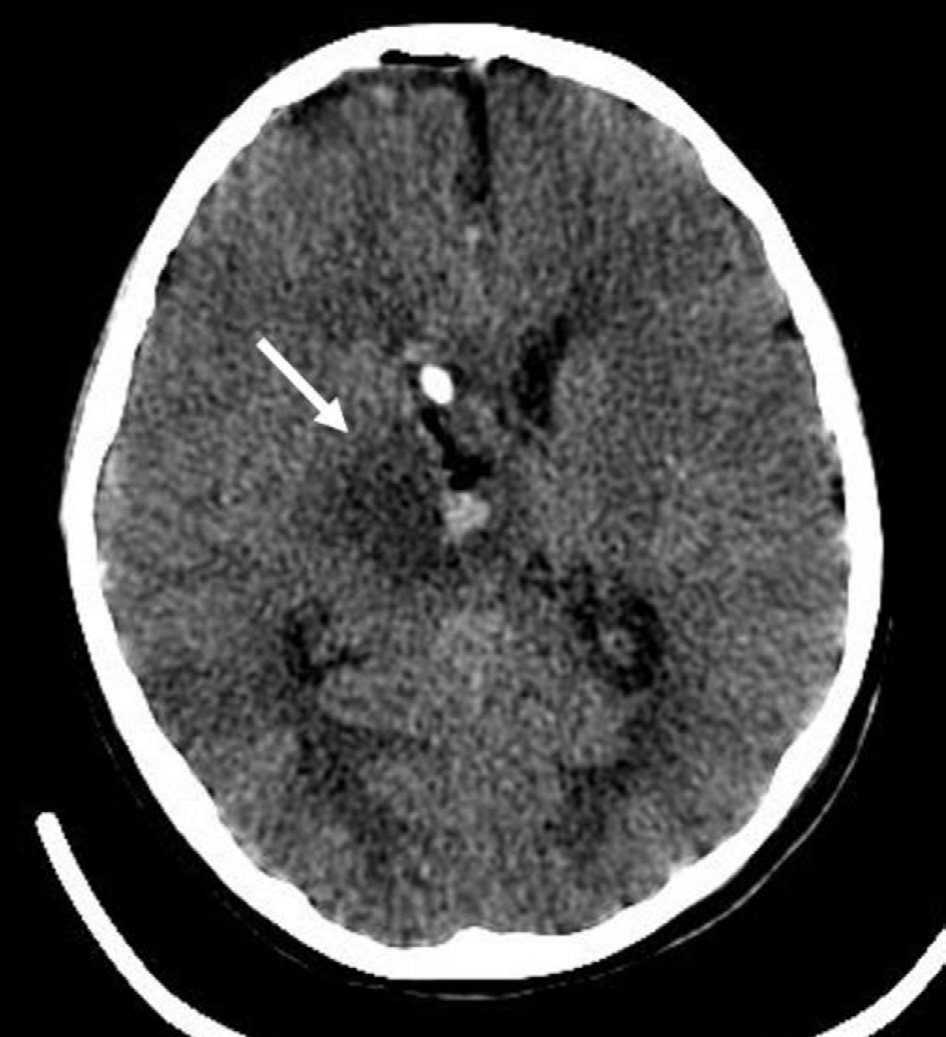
Postoperative computed tomography (CT) examination. The CT image shows that most of the tumours were resected and a little blood oozed in the operative area.
